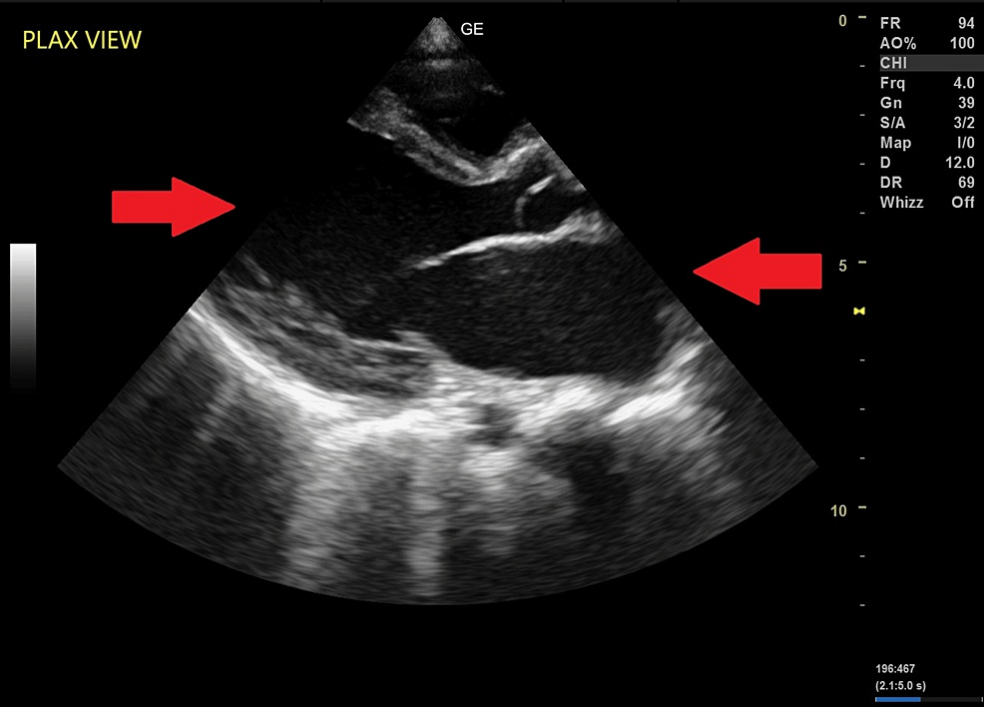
Ultrasound in the PLAX view showing dilated left atrium and left ventricle. PLAX, parasternal long axis
